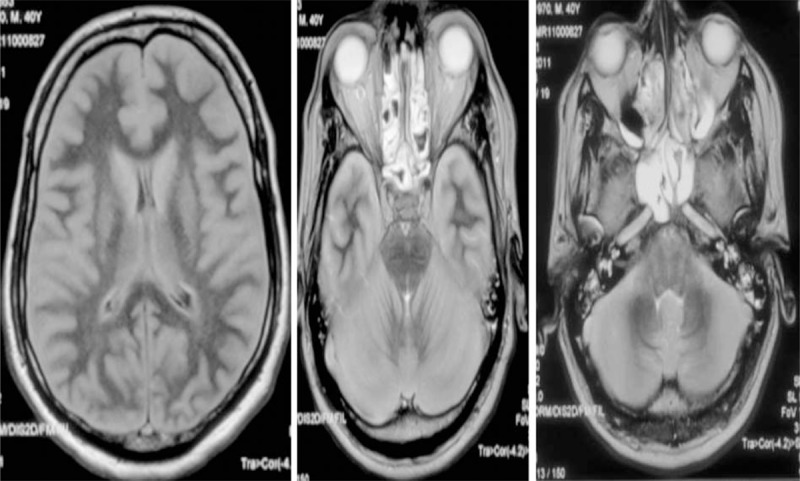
FLAIR MRI showing cerebral edema with thickening of the gray matter. Loss of ambient cistern and effaced 4th ventricle. FLAIR = fluid attenuated inversion recovery.
